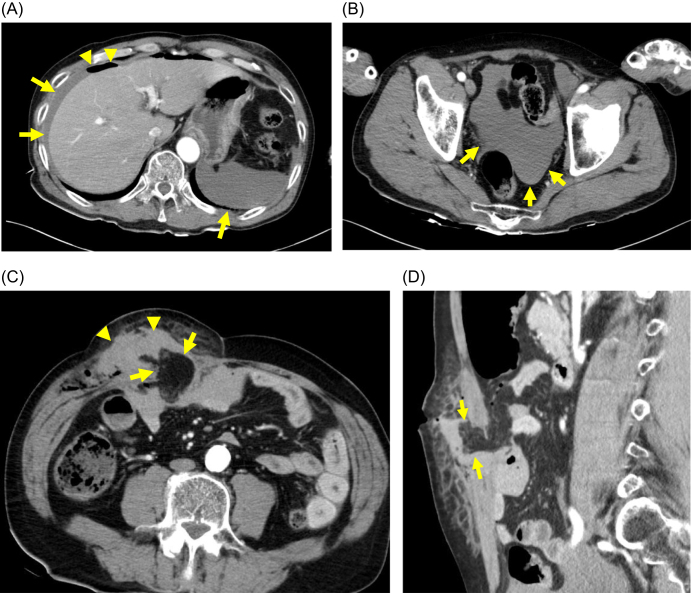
Preoperative computed tomography (CT) images. (A) Axial view showing intra-abdominal free air (arrowhead) and fluid collection (arrow). (B) Axial view showing fluid collection (arrow) in the pelvic space. (C) Axial view at the level of the stab wound showing a large abdominal wall hematoma (arrowhead) and the incarcerated omentum (arrow). (D) Sagittal view showing omental herniation through the fascial defect (arrow).
